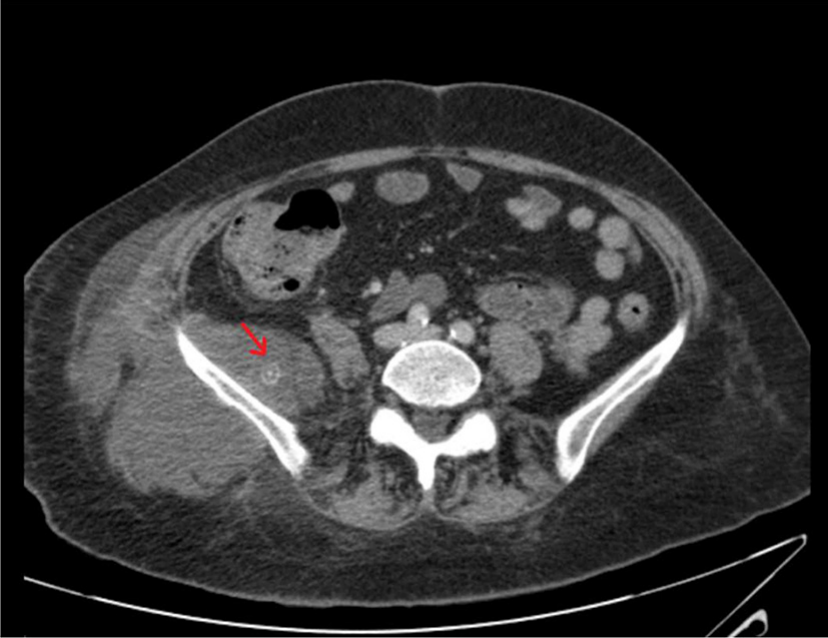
CT abdomen and pelvis (axial view) shows frank abscess with 9 mm well-defined lamellated calculus (red arrow).
